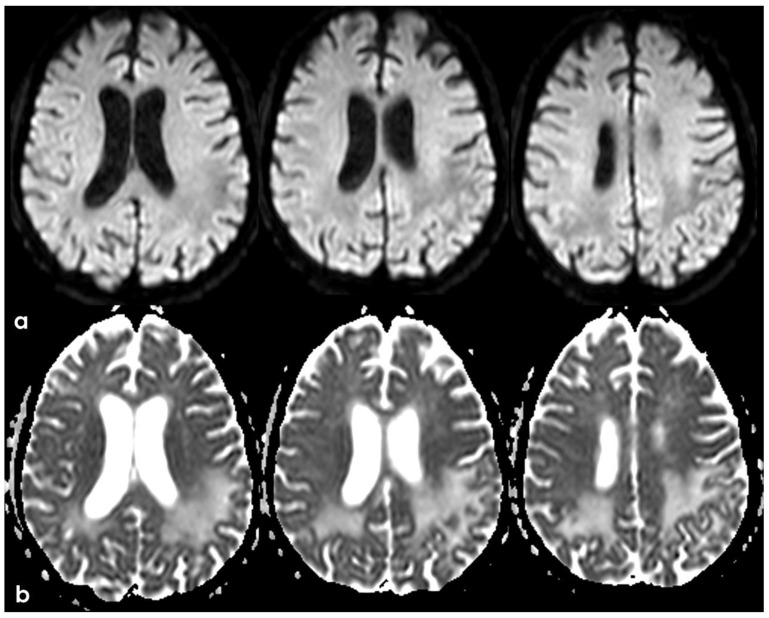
Brain MRI (axial DWI and ADC map) (panel (a) and (b), respectively), showing that the tumefactive white matter lesion shown in Figure 18 has features of vasogenic edema.
